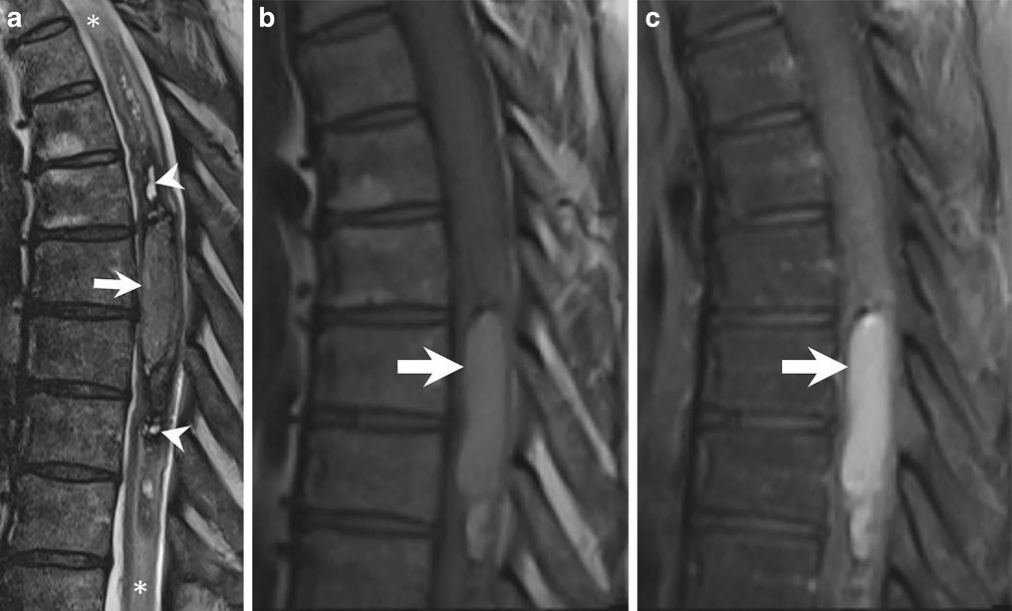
Sagittal T2-weighted MR images a show an intramedullary lesion extending from Th6 to Th11. The lesion consists of cystic areas with a hypointense rim (a, arrowheads), adjacent to a focal, space-occupying hyperintense portion at the Th8 level (a, arrow). Surrounding the lesion, a homogeneous centromedullary hyperintensity indicative of perifocal edema is observed (a, asterisks). On T1-weighted images prior to Gadolinium (Gd) administration, the space-occupying portion appears homogeneously hyperintense (b, arrow). Following Gd administration, T1-weighted images with fat saturation reveal no contrast enhancement within the lesion (c, arrow)
